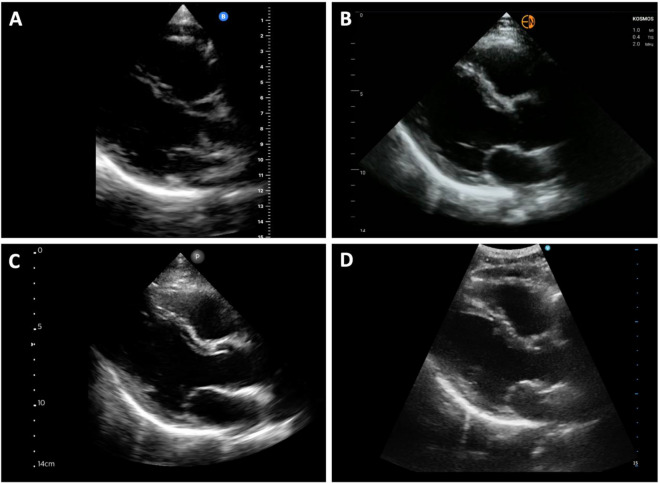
Parasternal long-axis view by four handheld ultrasound devices. Parasternal long-axis views acquired from the same standardized patient in early systole with the mitral valve closed and aortic valve open are shown from the A Butterfly IQ+™ (Butterfly Network, Inc.), B Kosmos™ (Echonous, Inc.), C Lumify™ (Phillips Healthcare), and D Vscan Air™ (General Electric)
